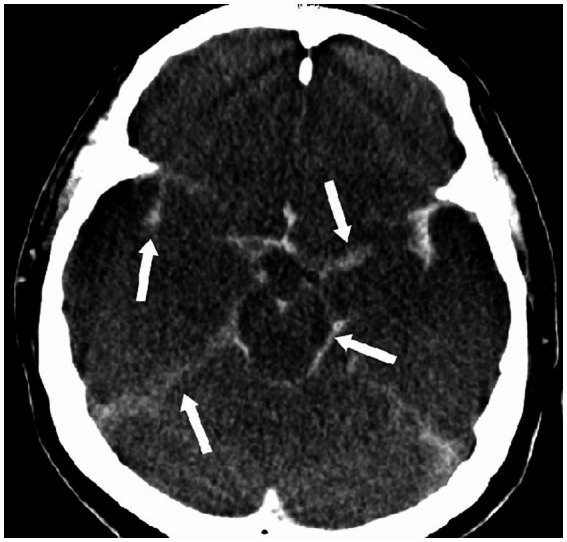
Pseudosubarachnoid hemorrhage. This 47-year-old man suffered from sudden cardiac arrest in a supermarket. Cardiopulmonary resuscitation was initiated by his friends until the arrival of paramedical professionals. Initially noted ventricular fibrillation was successfully cardioverted by two electric shocks, and he was intubated and brought to our emergency room (downtime 36 min). No brainstem reflexes were observed. The CT scan of the brain showed increased attenuation within the basal cisterns that mimics a subarachnoid hemorrhage.
